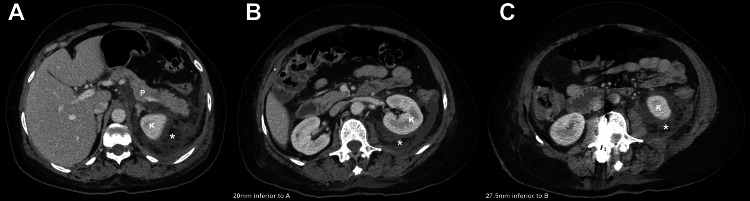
Axial CT images of our patient's abdomen. (A) The level near the T12-L1 disc space, and the tail of the pancreas can be seen. (B) The L2 level and is 20 mm inferior (i.e., closer to the sacrum) when compared to (A); the pancreas is not seen. Hence, her pancreas is mostly anterior to the L1 vertebral body. (C) The level of the L3 vertebral body and is 27.5 mm inferior to (B). Axial CT images were obtained six days after our patient’s lumbar spine surgery (November 20, 2022). Retroperitoneal fluid, indicated by the asterisk, can be seen near the left kidney. P: pancreas; K: kidney.
